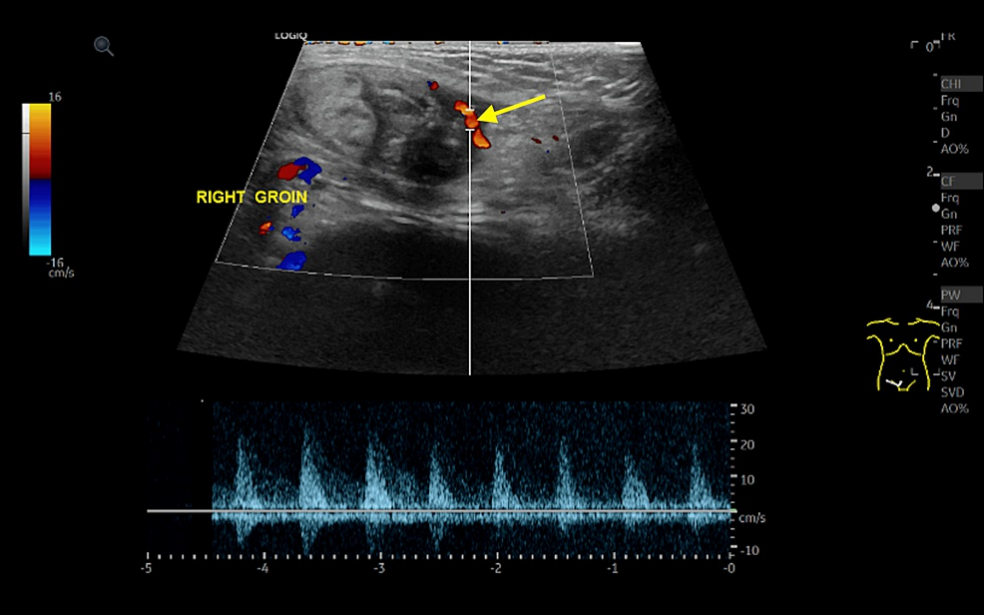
A doppler ultrasound image of the right groin shows an oval-shaped heterogeneous structure with peripheral vascularity (yellow arrow).
